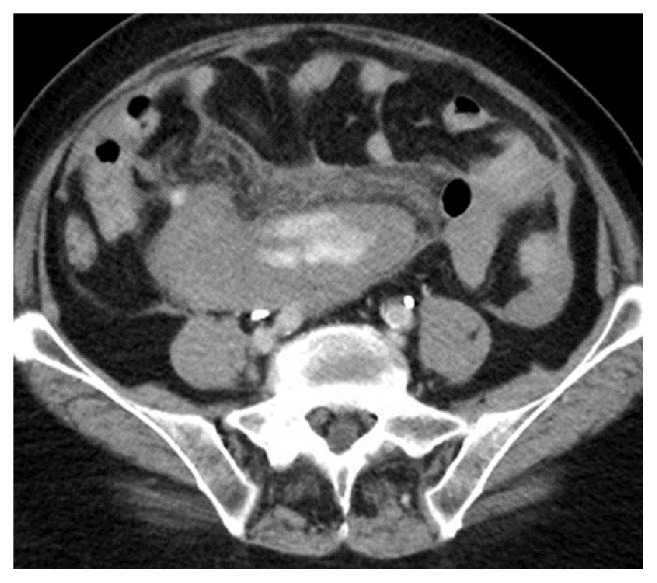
CTA abdomen showing hyperdense area of hemorrhage.
